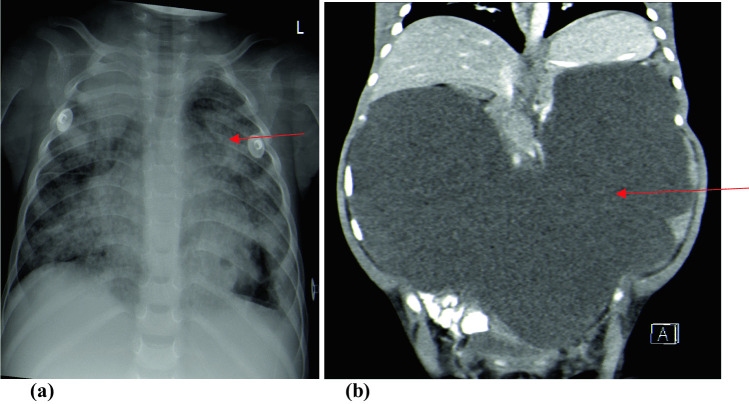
Chest X-ray of child with TB pneumonia (a) and abdominal CT showing ascites in a child diagnosed with TB abdomen (b)
